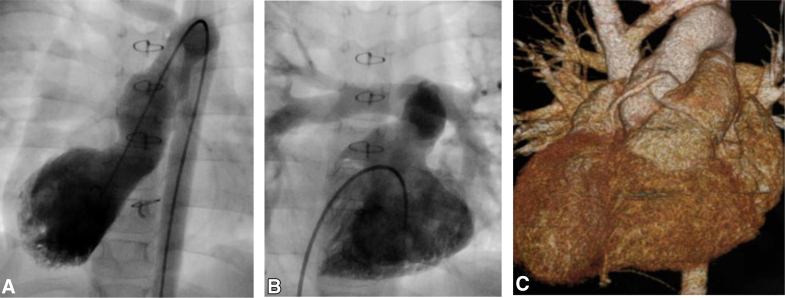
Postoperative catheterization and computed tomography scanning. A, Left ventricle angiography showed that the left ventricle outflow tract was straight. B and C, Right ventricle angiography and computed tomography showed that the conduit was on the left of the sternum, avoiding sternal compression.
